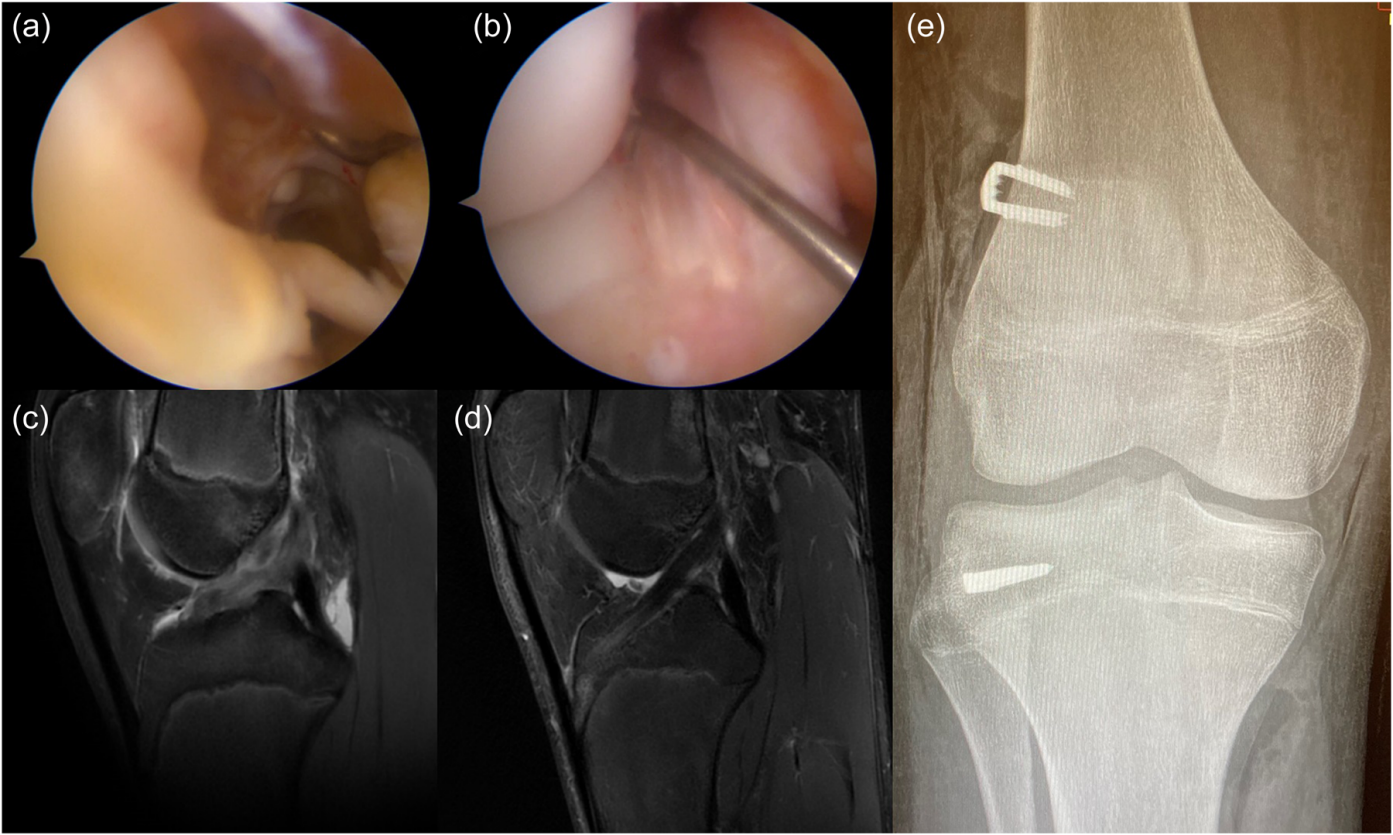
A male football player with chronological age of 14.2 years and skeletal age of 14 years was treated with supra‐physeal over‐the‐top ACL reconstruction. Arthroscopic vision with the empty notch due to ACL injury (a) and after reconstruction with hamstring graft (b). Sagittal pre‐operative MRI shows the absence of the ACL (c) and 6‐month MRI shows the graft with a good ipointense signal and the course above the growth plate and within the proximal epiphysis (d). Antero‐posterior radiograph shows no conflict of the staples with the growth plates (e). ACL, anterior cruciate ligament.
